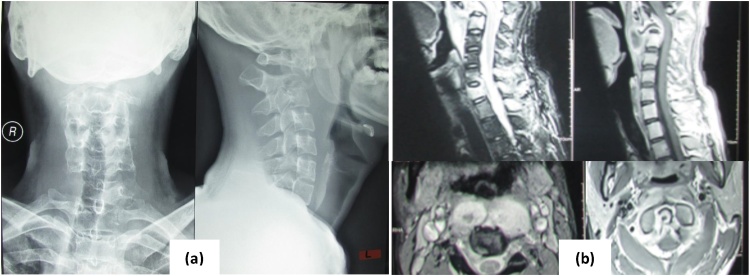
Radiology examination. (a) X-ray Cervical AP and Lateral. (b) MRI cervical sagittal and axial.
